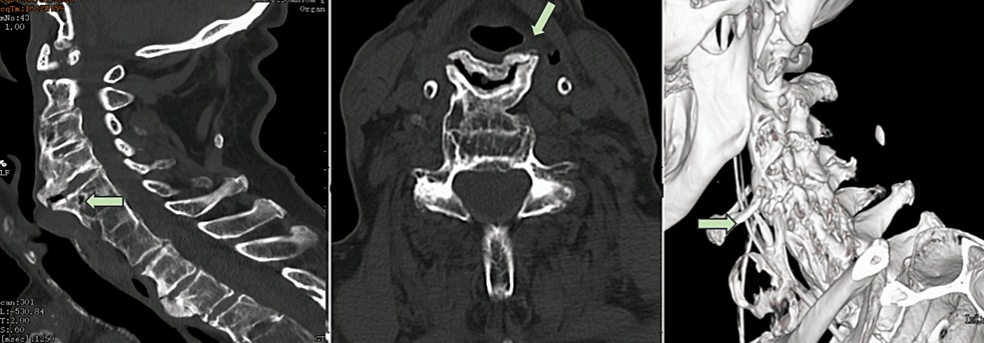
Preoperative CT imaging showing exuberant anterior C4-C5 osteophyte comprising the posterior hypopharyngeal wall. CT, computed tomography
